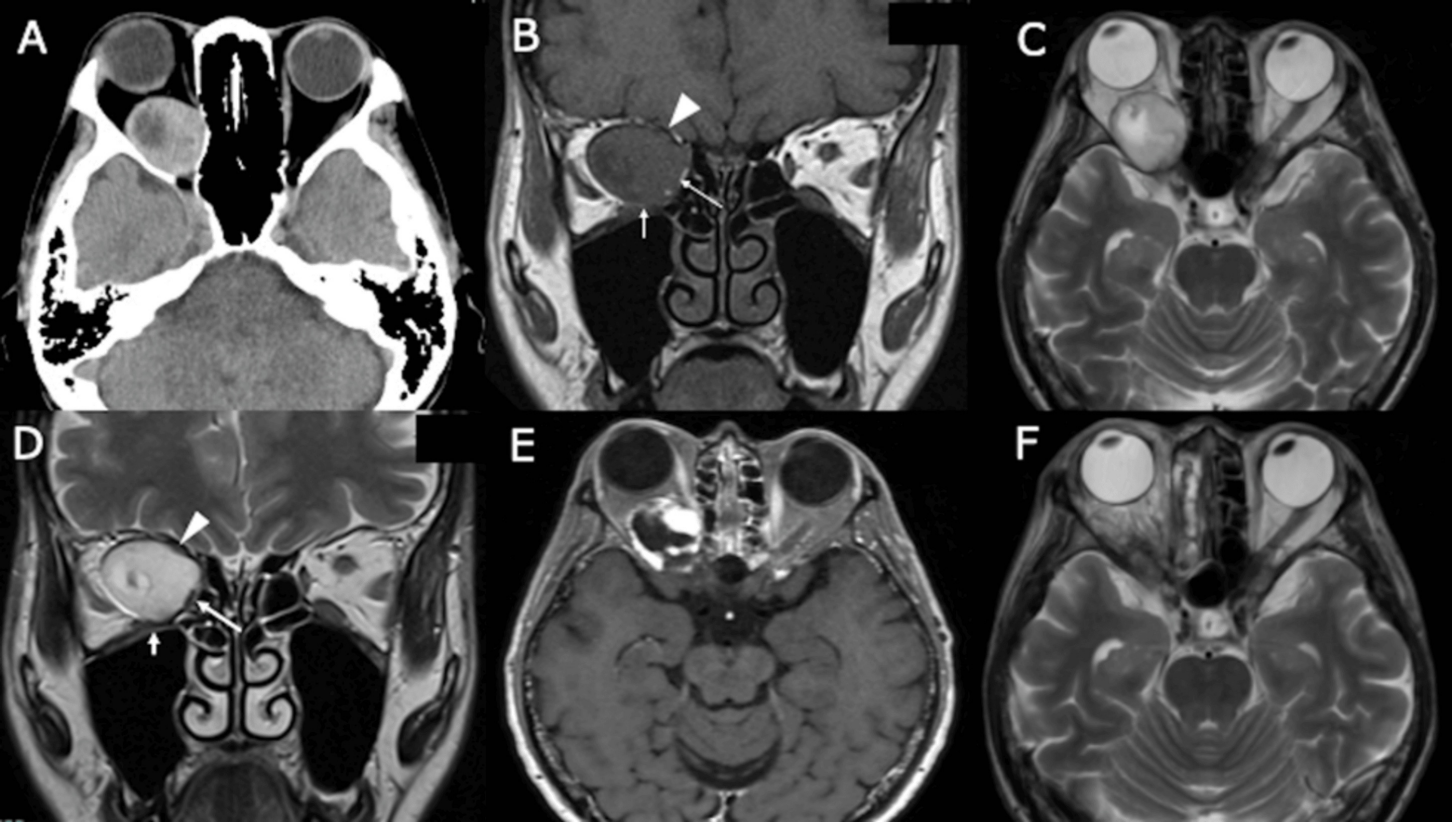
Radiological images (A) Preoperative axial cranial CT scan showing an isodense retrobulbar mass in the right orbit, causing anterior displacement of the globe. (B-E) Preoperative MRI demonstrating an oval-shaped intraorbital lesion with isointensity on T1-weighted imaging (B) and hyperintensity on T2-weighted imaging (C, D). Coronal views (B, D) show compression and thinning of the medial rectus muscle (long arrow) and inferior rectus muscle (short arrow), with a narrow intermuscular corridor between them. The lesion displays heterogeneous delayed contrast enhancement in the late phase (E). The optic nerve (arrowhead) is compressed superiorly and appears thinned. (F) Postoperative MRI confirming total resection of the lesion and resolution of exophthalmos.
